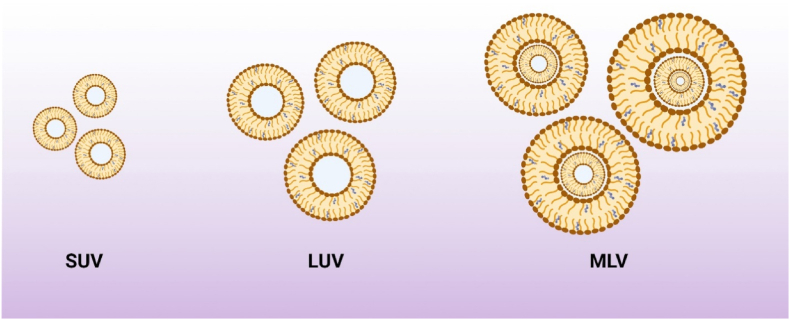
Types of niosomes based on the size and number of lamellar. SUV: small unilamellar vesicles, LUV: large unilamellar vesicles, MLV: multilamellar vesicles.
